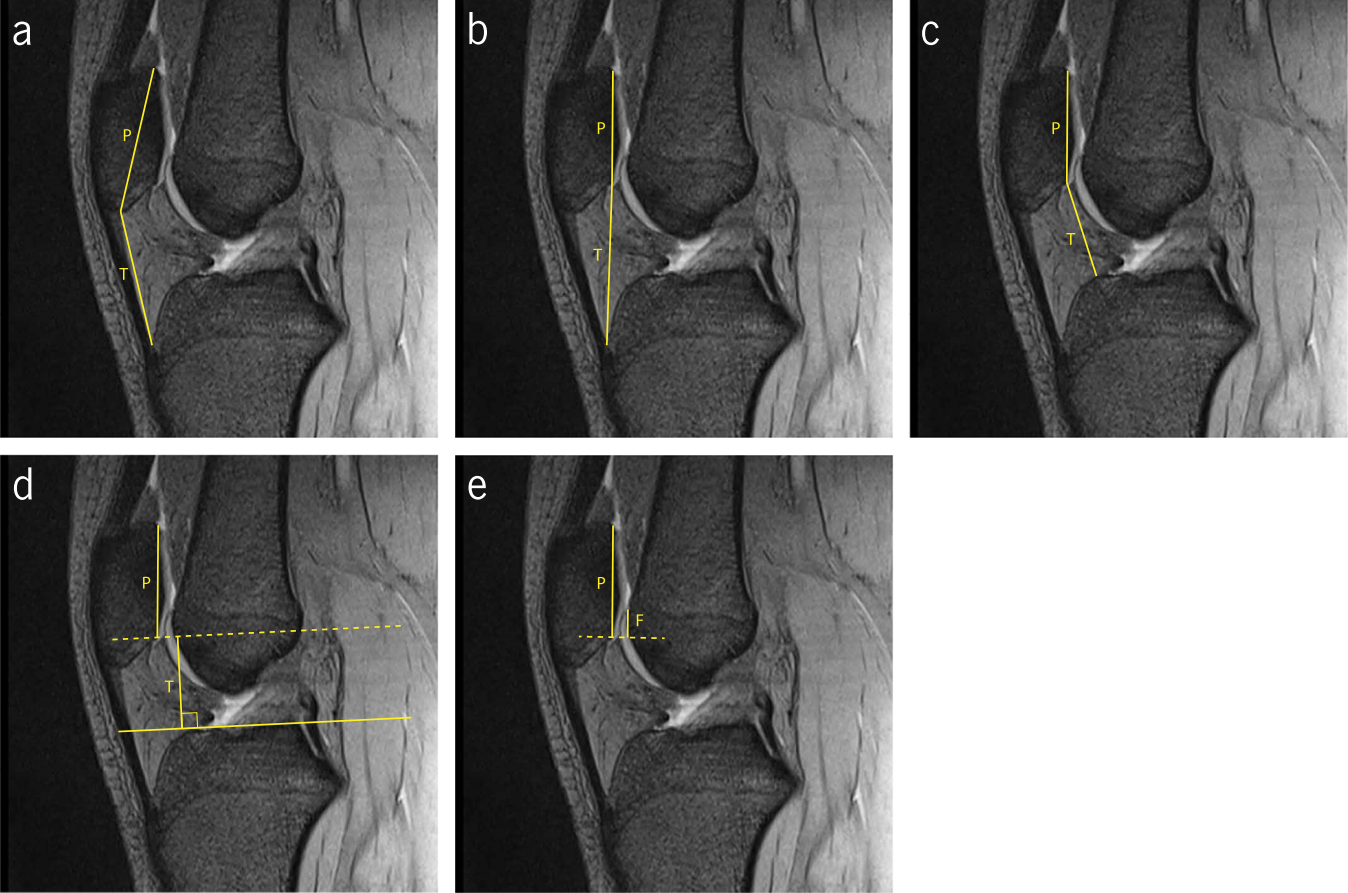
Sagittal gradient echo T2-weighted magnetic resonance images demonstrating the measurements of the indices to assess patellar height. a Insall–Salvati Index (T/P). b Modified Insall–Salvati Index (T/P). c Caton–Deschamps Index (T/P). d Blackburne–Peel Index (T/P). e Patellotrochlear Index (F/P)
